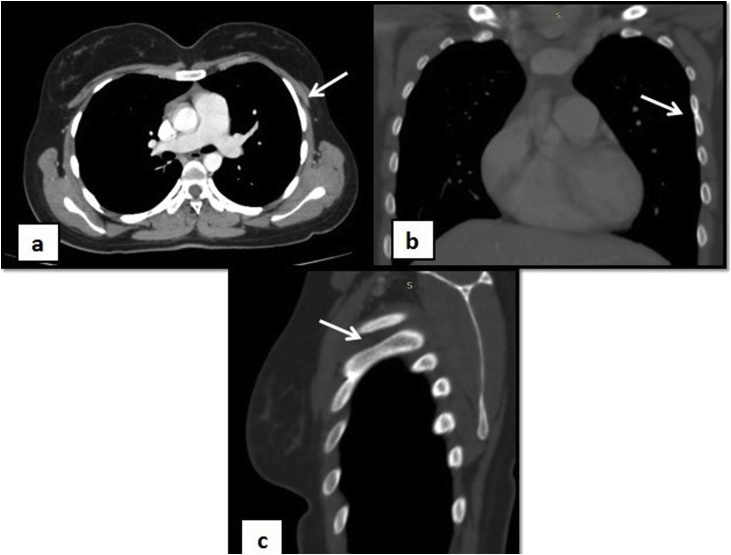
Contrast enhanced Computed tomography (CT) chest shows normal bilateral breast tissue with bifid rib on left.
